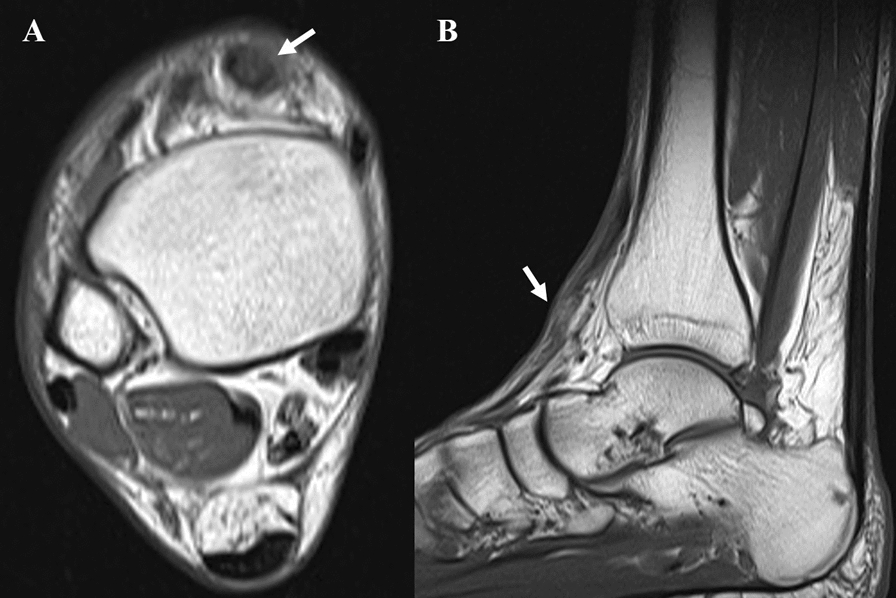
Preoperative magnetic resonance imaging (MRI). A Axial and B sagittal views showing bulbous expansion of the tibialis anterior tendon at the level of the talocrural joint (white arrows), consistent with degenerative rupture
